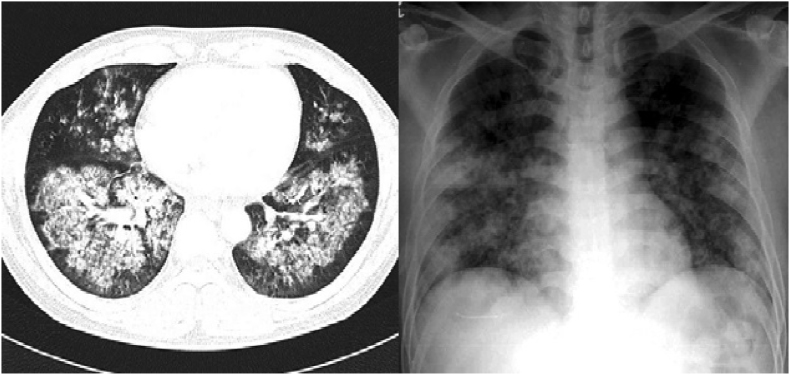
Chest radiography and CT confirmed the pre-existing chronic obstructive pulmonary disease. Chest CT showed parenchymal infiltrates through both lung fields on admission. Chest radiography showed patchy consolidation infiltration in both lung fields on admission.
